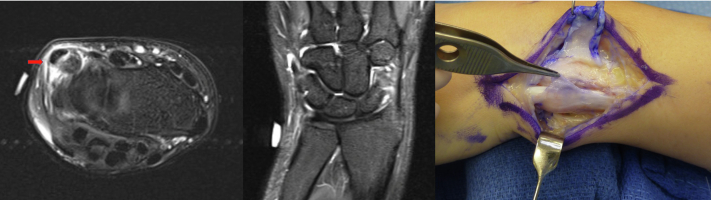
MRI and intraoperative findings showing a chronically attenuated Inoue and Tamura type C (Allende and Le Viet type B) ECU subsheath injury with concurrent ECU tenosynovitis.
